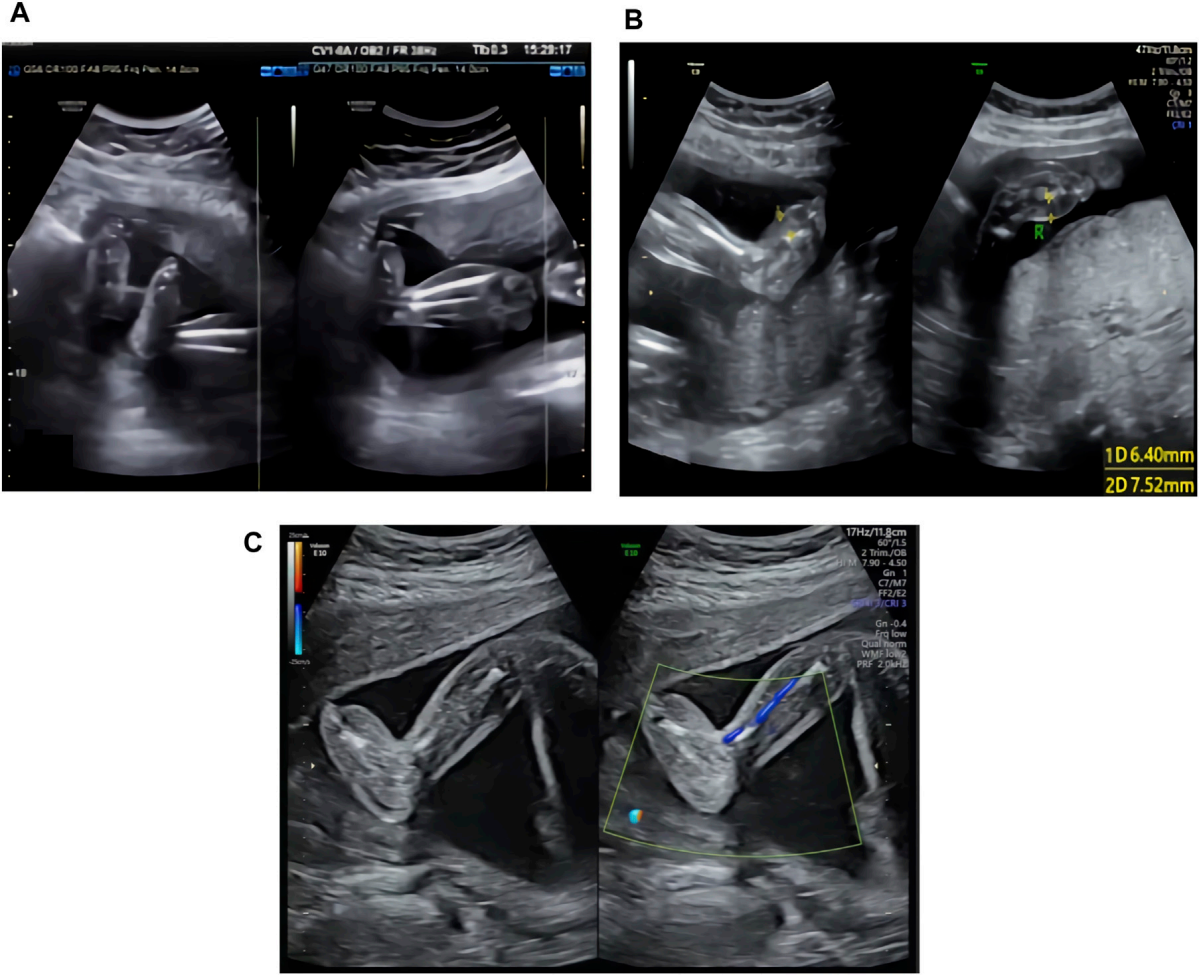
(A) Ultrasound image of the family 1 fetus at 22 WG: thickening of the subcutaneous tissues in both lower legs and feet. (B) Ultrasound image of the family 2 fetus at 24 WG: edema in both feet and foot dorsum skin thickening. (C) Ultrasound image of the family 3 fetus at 23 WG: thickening of subcutaneous tissues in both feet.
